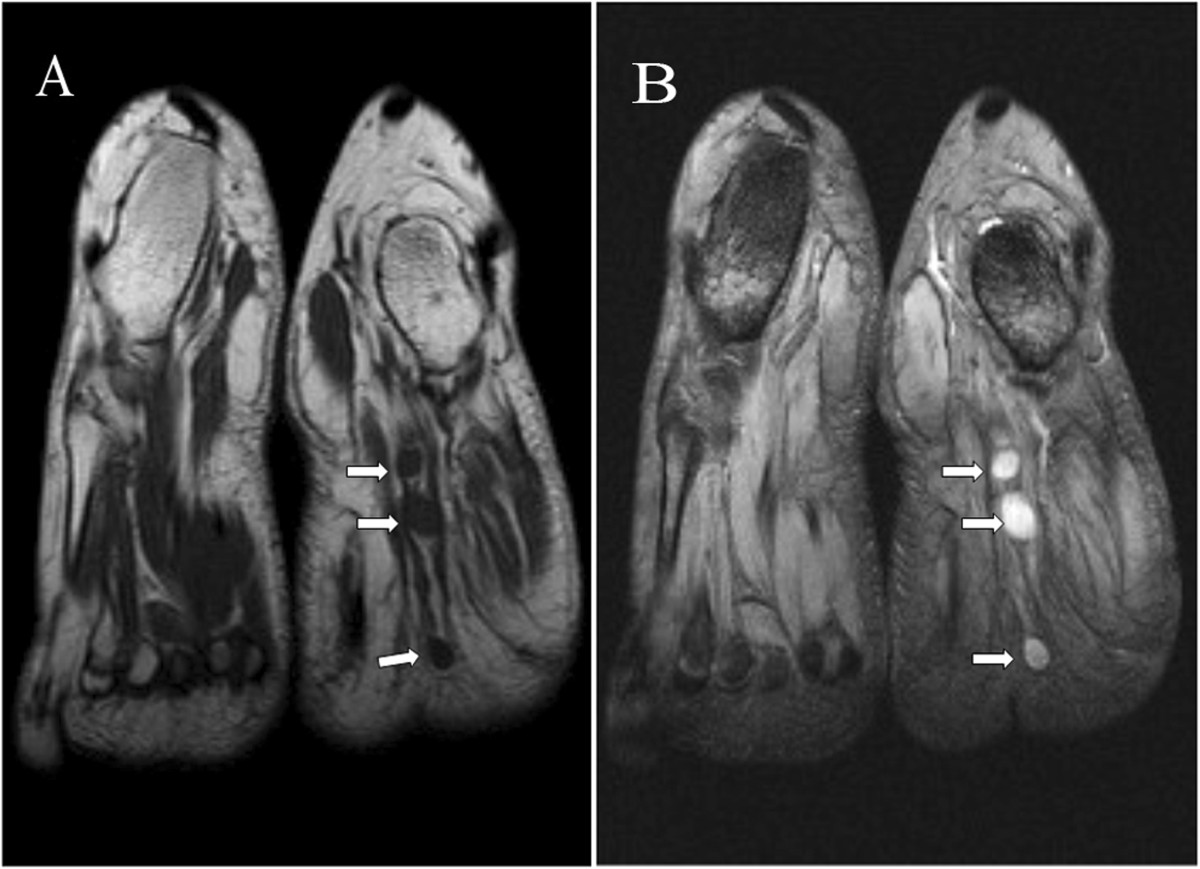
Coronal MR images showing linear round lesions. (A): T1-weighted fast-spin echo image (TR/TE: 512/11) shows the lesions with homogeneous isointensity to skeletal muscle (*arrows*). (B): T2-weighted multi echo data image combination sequence (TR/TE: 865/23) demonstrates three prominent nodules of high intensity signal (*arrows*).
